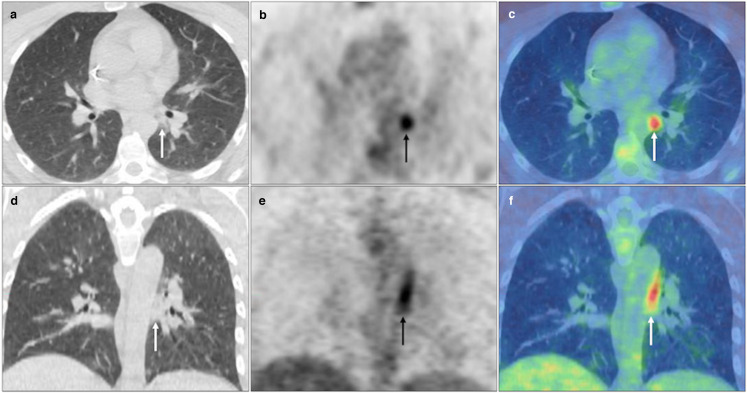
Unilateral FDG avid (SUV_max_ 7.0) vertically orientated consolidation posterior to the left lower lobe bronchus (solid black and white arrows) on axial CT, PET and fused PET-CT (A-C, D-F). BSTI 3 with positive RT-PCR (*confirmed* case).
